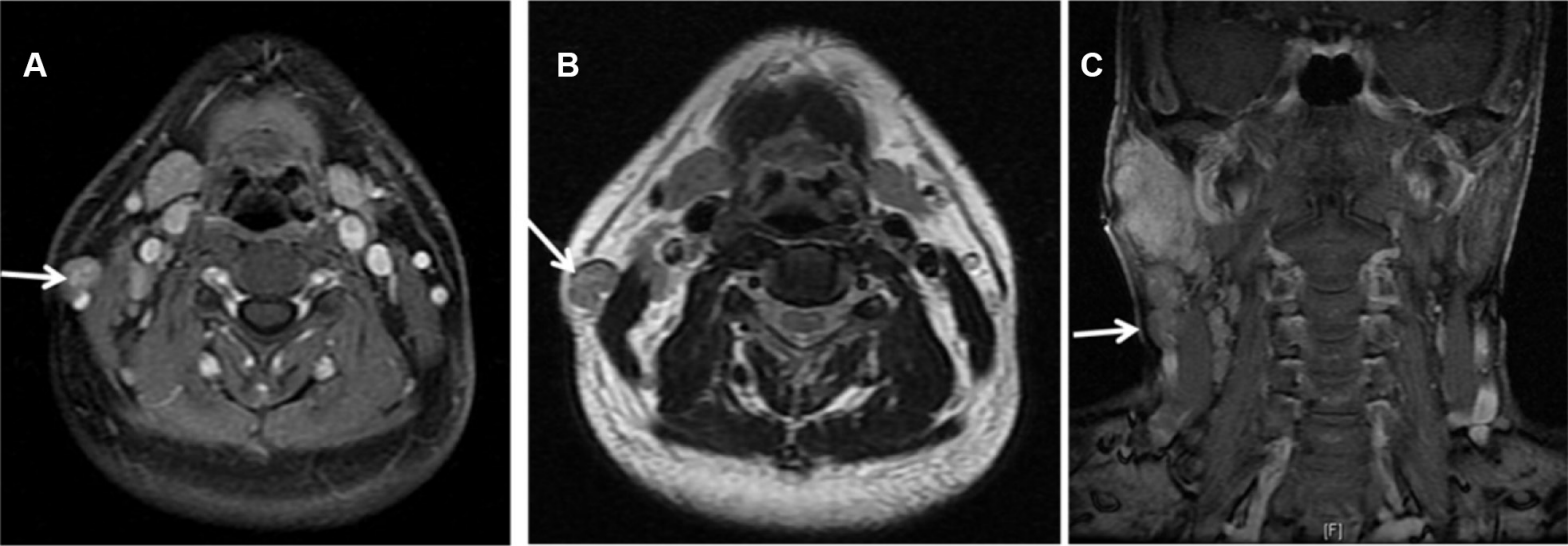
MRI showing nodal metastasis (arrow) at the surface of the right sternocleidomastoid muscle in a 51 years old male patients with LELC of the right parotid (A) Contrast enhanced transverse T1WI MRI with fat suppression. (B) Transverse T2WI MRI. (C) Contrast enhanced coronal T1WI MRI with fat suppression.
